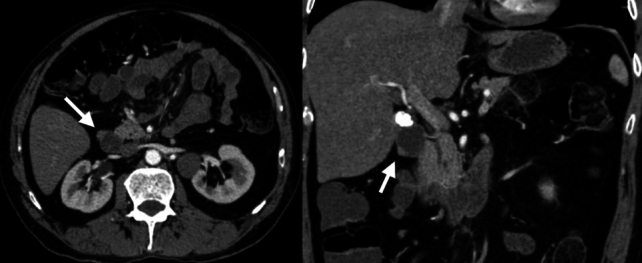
Axial and coronal negative oral and IV contrast-enhanced CT images of a 57-year-old male patient. A nodular lesion with density and enhancement characteristics similar to pancreatic parenchyma is observed, consistent with ectopic pancreatic tissue (arrows)
